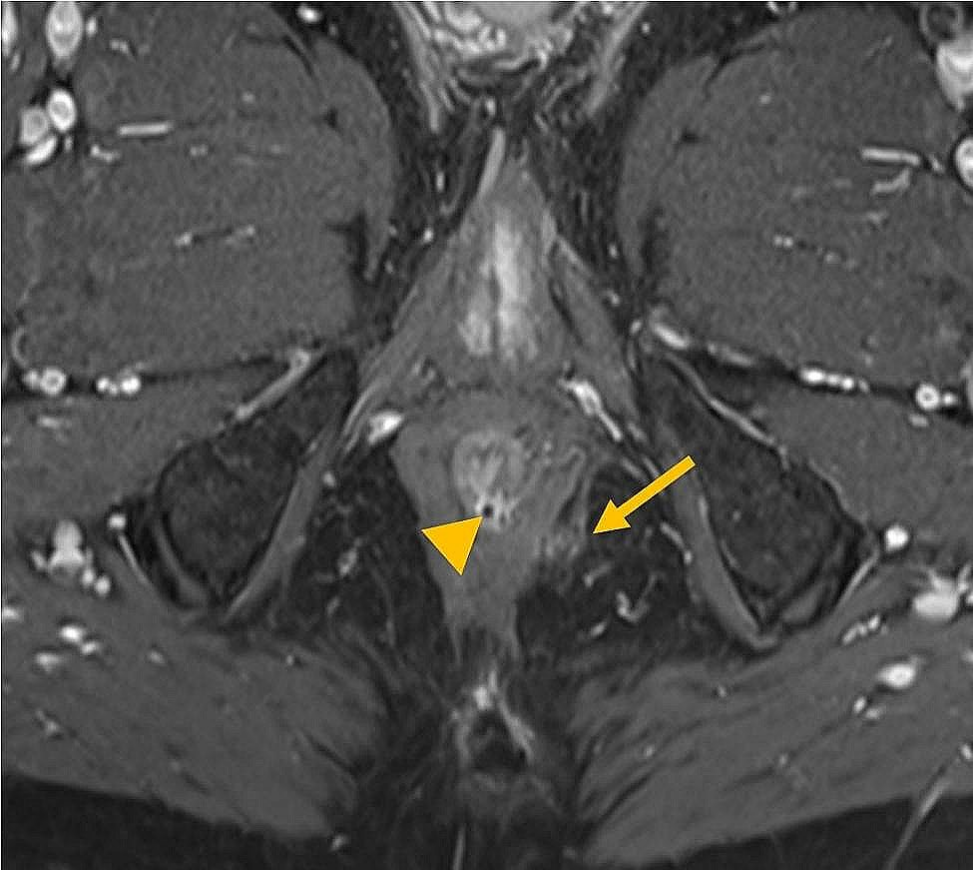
b. Post-treatment MRI: An axial T1-weighted sequence with fat suppression after contrast administration with a total MAGNIFI-CD score of 5 based on a single fistula tract with a fistula length between 2.5 and 5 cm, without extension, mild T1 hyperintensity and more than 50% fibrotic tissue. Artefact in the posterior intersphincteric space (arrow head) caused by the MSC treatment
